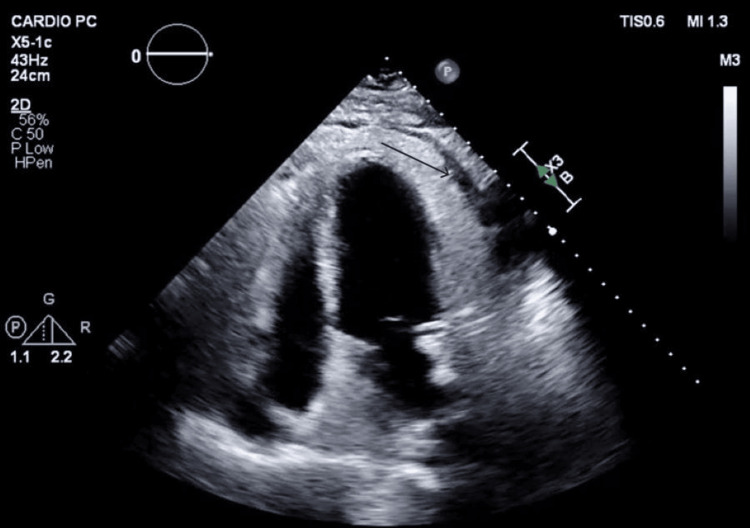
Apical two-chamber window showing moderate pericardial effusion (black arrow)
